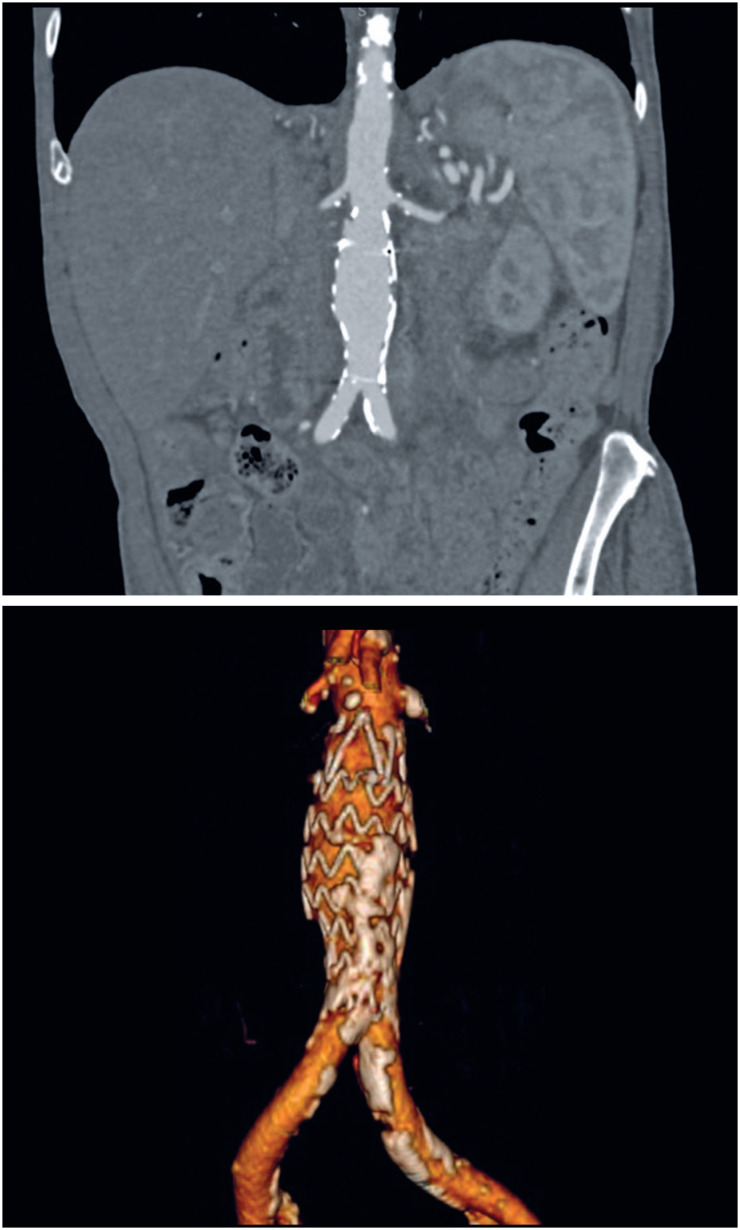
Computed tomography performed 6 months after surgery. Total exclusion of the pseudoaneurysm and absence of endoleaks. No migration of the endoprosthesis is demonstrated
